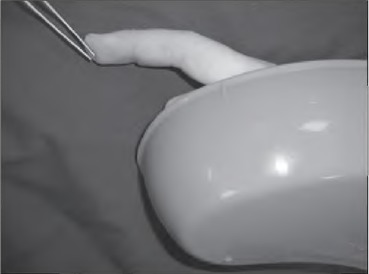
Kidney dish used to produce an image similar to the x-ray in Figure 1
